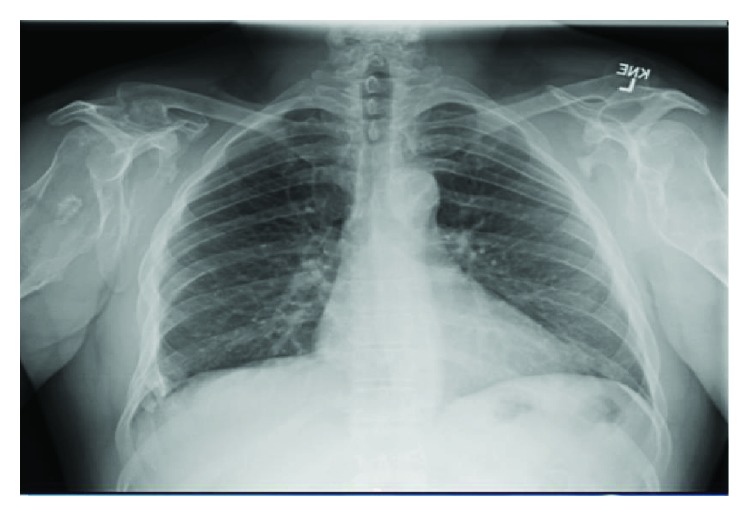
An AP X-ray view displaying multiple osteochondromas at multiple sites in the clavicles, scapulae, and proximal humeri bilaterally as well as multiple right inferior anterior ribs.
